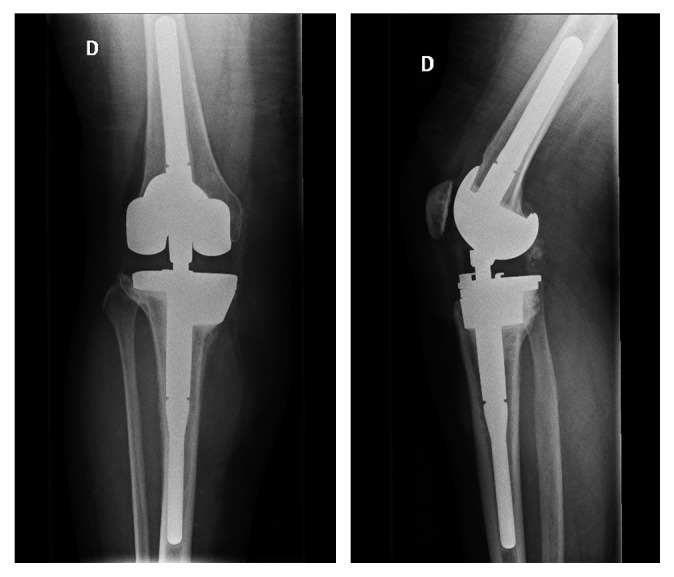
X-rays showing 2 years postoperatively rotating hinge total knee replacement with metal augment.
